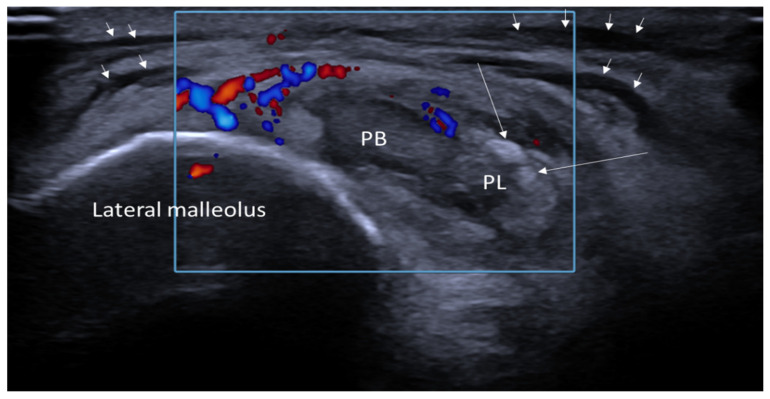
Axial ultrasound images of the ankle joint in a patient with gout, focusing on the peroneal tendons. The images reveal characteristic hyperechoic formations (long arrows), consistent with monosodium urate (MSU) deposits, representing an extra-articular manifestation of gout. Additionally, diffuse hypoechoic areas are visible in the subcutaneous tissue (short arrows), indicating the presence of lymphedema. PB: peroneus brevis tendon, PL: peroneus longus tendon.
